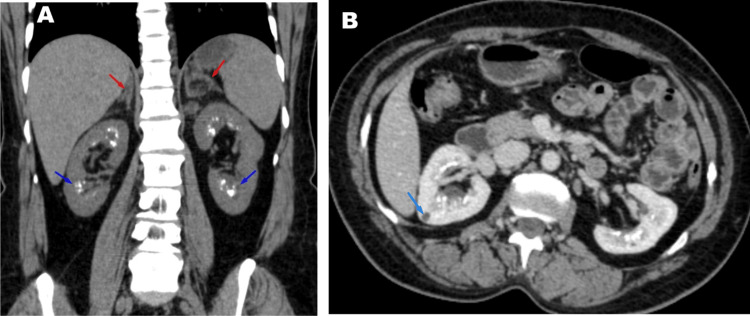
CT scan findings of Case 2: non-classic 11-beta hydroxylase deficiency A: Bilateral adrenal hyperplasia with myelolipoma (red arrow). Medullary nephrocalcinosis (blue arrow) B: Renal cyst with medullary nephrocalcinosis
